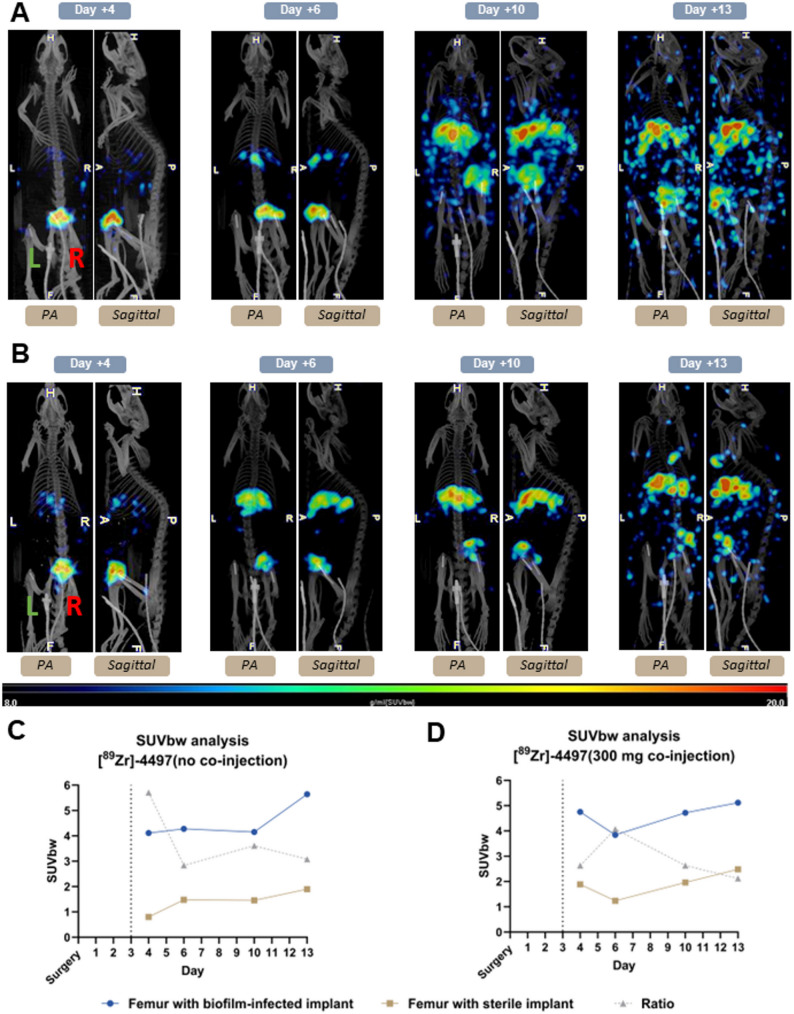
The ImmunoPET tracer shows specific targeting of the infection throughout the study duration. The green L indicates the left sterile implant and the red R indicates the right biofilm-infected implant. Posteroanterior (PA) and sagittal planes are depicted. Dotted line indicates the day of injection. (a) PET/CT scans of the [^89^Zr]−4497 with no co-injection of cold 4497-antibody. (c) PET/CT scans of [^89^Zr]−4497 (ImmunoPET) with no co-injection show five times more accumulation of the ImmunoPET tracer in the femur with biofilm-infected implant compared to the femur with sterile implant at day four post-surgery. The ratio SUVbw between the femur with biofilm-infected implant and femur with sterile implant on days 4, 6, 10 and 13 post-surgery was 5.71, 2.84, 3.61 and 3.08, respectively. (b) PET/CT scans of the [^89^Zr]−4497 with 300 µg co-injection of cold 4497-antibody. (d) The ratio SUVbw between the femur with biofilm-infected implant and femur with sterile implant on days 4, 6, 10 and 13 post-surgery was 2.63, 4.07, 2.63 and 2.12, respectively
